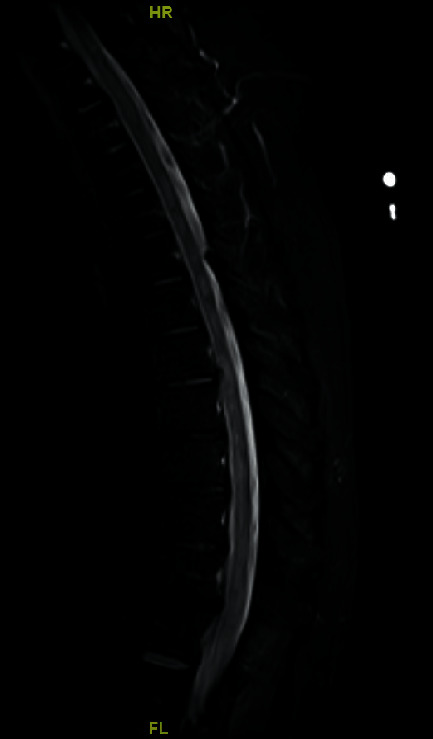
MRI spine T2-weighted sagittal image showing evidence of longitudinally extensive transverse myelitis (LETM).
